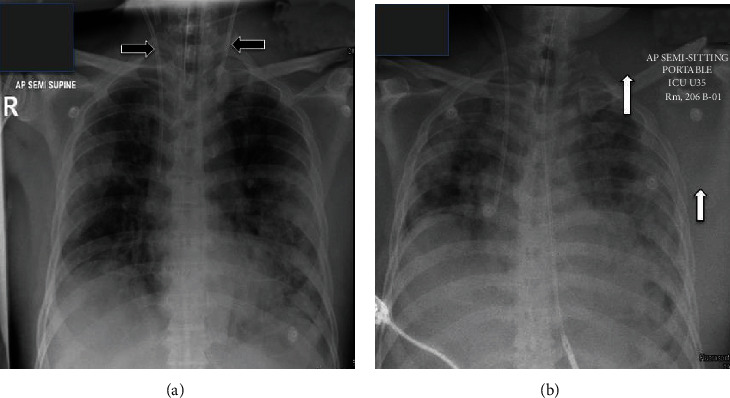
(a) Chest X-ray showing two double lumen hemodialysis central vascular access. (b) Chest X-ray after the removal of the one on the left side with significant soft tissue swelling around the left shoulder associated with left lateral displacement of the scapula.
